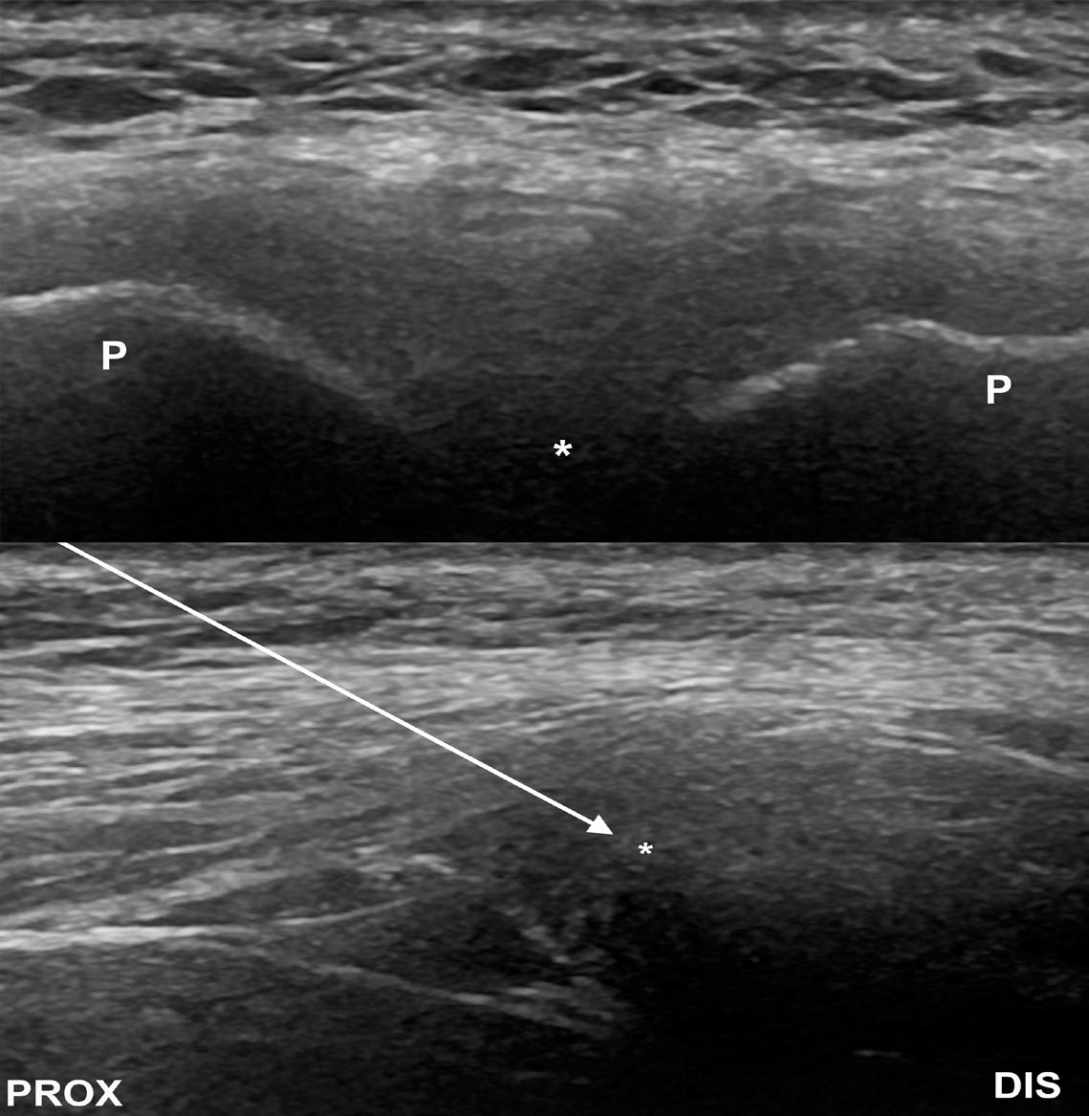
(A, image above) Transverse image of the symphysis pubis. P, pubis. (B, image below) Sagittal ultrasound image for symphyseal injection. The needle is introduced from a superior approach (arrow). Asterisk indicates the joint. DIS, distal; PROX, proximal.
